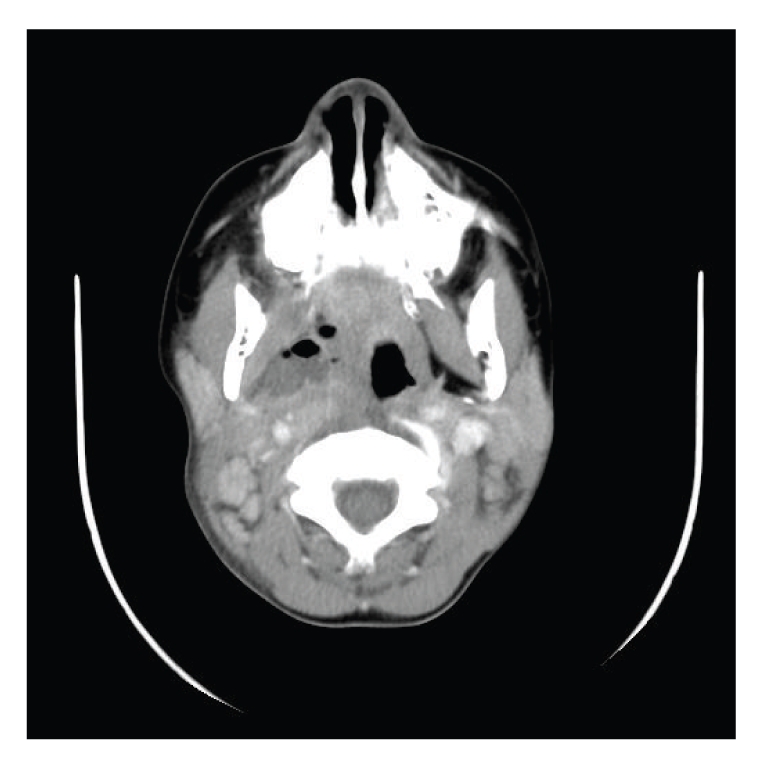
A CT scan with contrast showing compression of right internal jugular vein.
